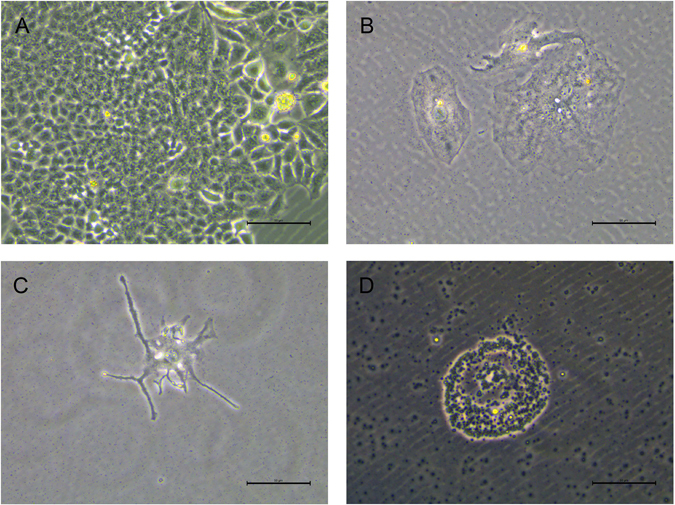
X-ray irradiation induced death in HepG2 cells *in vitro*. After 0 Gy (A), 30 Gy (B) and 50 Gy (C) X-rays irradiation, HepG2 cells were cultured for 7 d. HepG2 cells were cultured for 14 d after exposure to 30 Gy X-ray irradiation (D). Scale bar = 50 μm.
